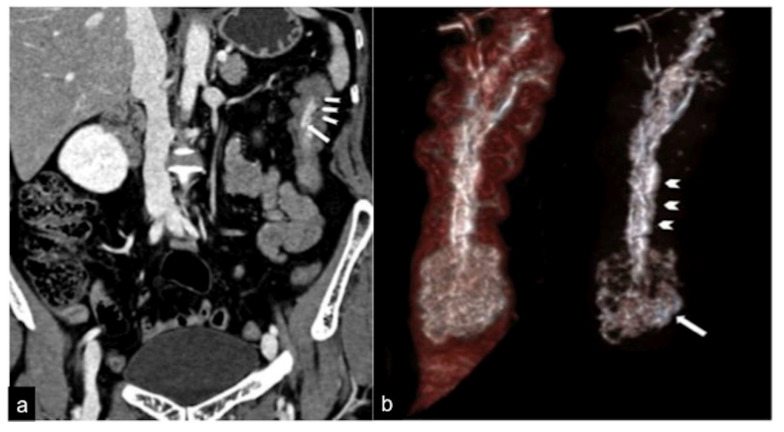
CTA coronal reconstruction in a patient with sub-occlusive recurrent status and occult haemorrhage. The coronal reconstruction (a) detects a pedunculated polyp (long arrow) with an arterial axis of vascular support. In the MIP post-processing with MPR and VR-3D, the coronal view (b) better defines the polypoid formation and the axis of vascular support (head arrows). Adopted with permission from ref. [15].
